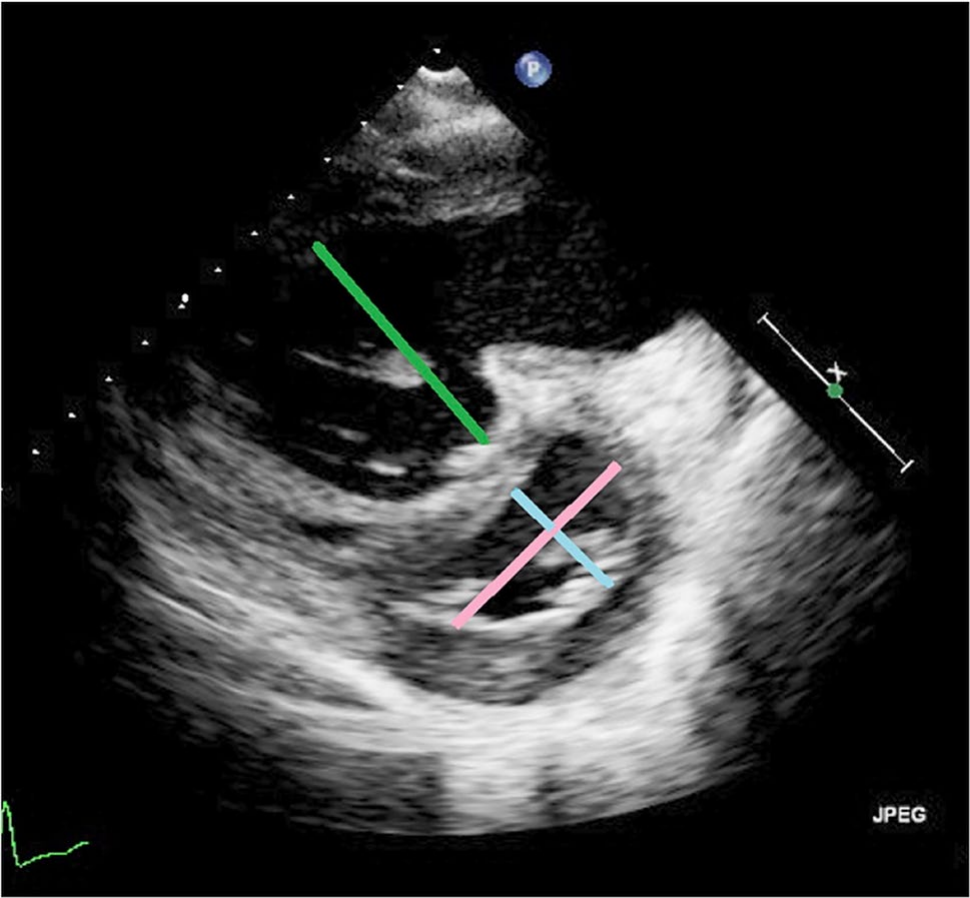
Measurement of LVEI and RV/LV ratio. End-systolic LVEI is measured as the ratio of the LV dimension parallel to the ventricular septum (pink) to the LV dimension perpendicular to the ventricular septum (blue) in parasternal short axis view. Systolic RV/LV ratio is measured as the ratio of the RV dimension (green) to the LV dimension perpendicular to the ventricular septum (blue) in the parasternal short axis view
